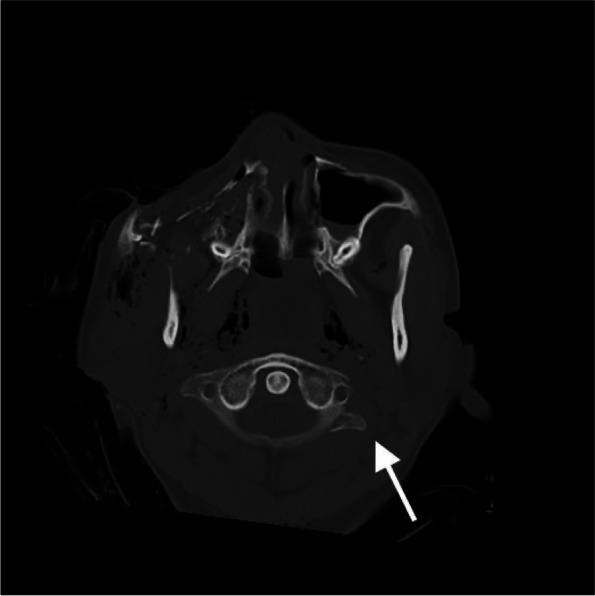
Cervical spine computed tomography scan: C1 cervical transverse fracture (arrow)
